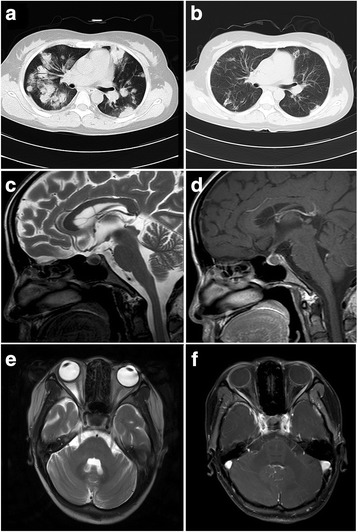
Magnetic resonance imaging (MRI) data. a Chest computed tomography before treatment; b Chest computed tomography after treatment. c Round signal (8 × 6 mm) with a clear edge on the posterior pituitary. d Mild dynamic contrast-enhancement in the delayed phase. e Axial T2 MRI showing the optic nerve cut. f T1, postcontrast MRI with fat suppression showing the optic nerve cut
